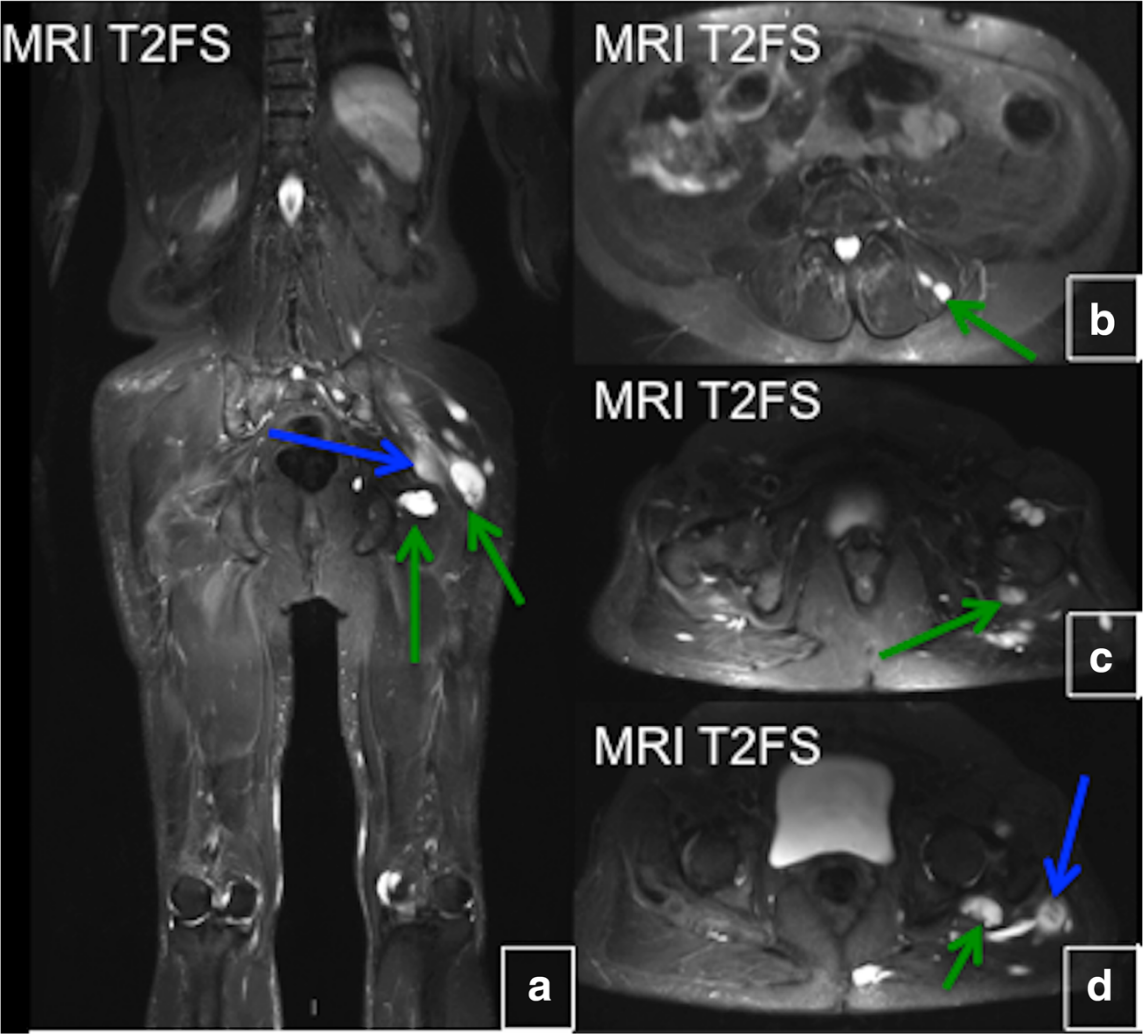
a–d Intramuscular myxomas in Mazabraud syndrome. Sagittal and axial T2-weighted fat-saturated magnetic resonance images demonstrate several high-signal-intensity lesions (*green arrows*). Some of the lesions show peripheral oedema (*blue arrows*)
